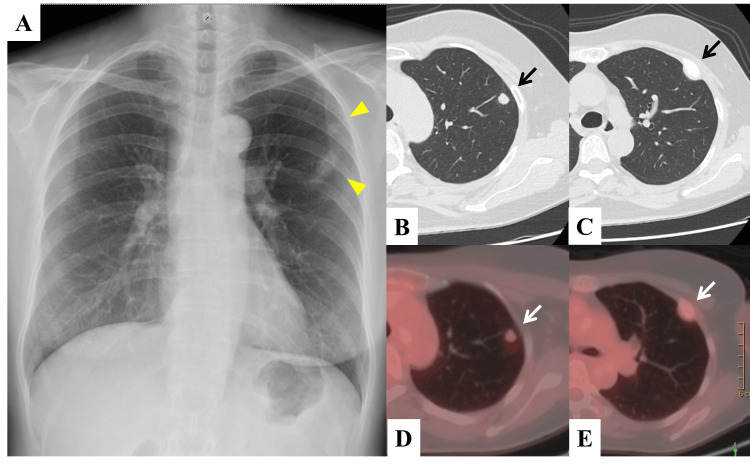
Clinical images of Case 1 (A) Chest radiograph showing two well-defined masses in the left upper pulmonary field (two arrowheads). (B and C) Chest computed tomography (CT) showing a mass measuring 9 x 7 mm in size in the left upper lobe S1+2 (arrow) and a soft tissue lesion in the left third rib (arrow). (D and E) An 18F-fluorodeoxyglucose (FDG)-positron emission tomography (PET)/CT showing accumulation in each lesion (SUVmax=1.66 and 1.96) (white arrows). SUVmax: maximum standardized uptake value
